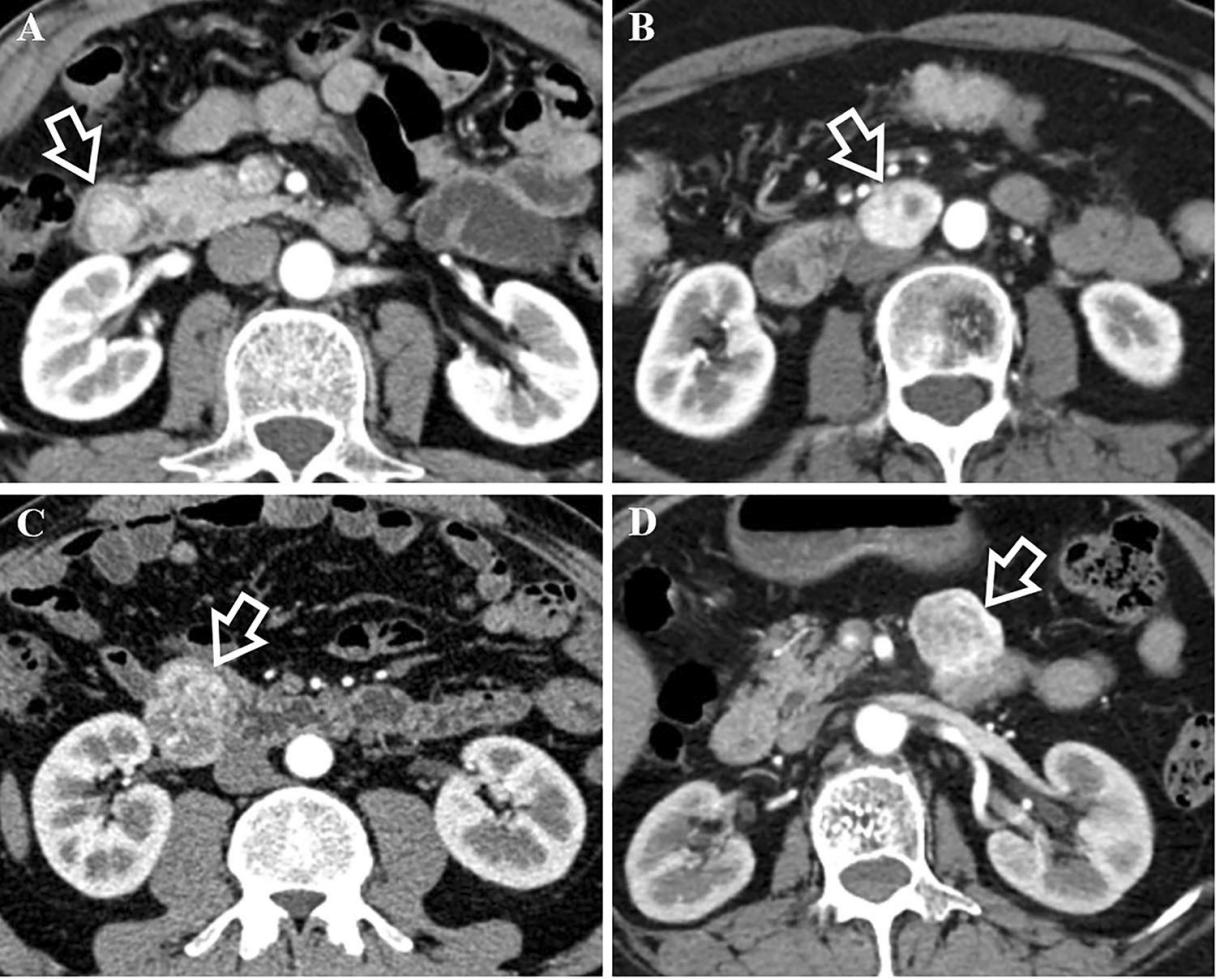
Comparison of growth pattern and intratumoral vessels in the arterial phase between dNENs (A, B) and dGISTs (C, D). A 57-year-old man with a G1 dNEN. CT scan shows an intraluminal nodule (white arrow) lack of intratumoral vessels in the descending part of the duodenum. B 59-year-old female with a G2 dNEN. There is a lesion (white arrow) lack of intratumoral vessels and presenting extraluminal growth pattern in the horizontal part of the duodenum. C 63-year-old male with a dGIST. It shows a lesion (white arrow) containing intratumoral vessels with mixed growth pattern in the descending part of the duodenum. D 66-year-old female with a dGIST. CT scan shows a lesion (white arrow) containing intratumoral vessels and presenting extraluminal growth pattern in the ascending part of the duodenum
